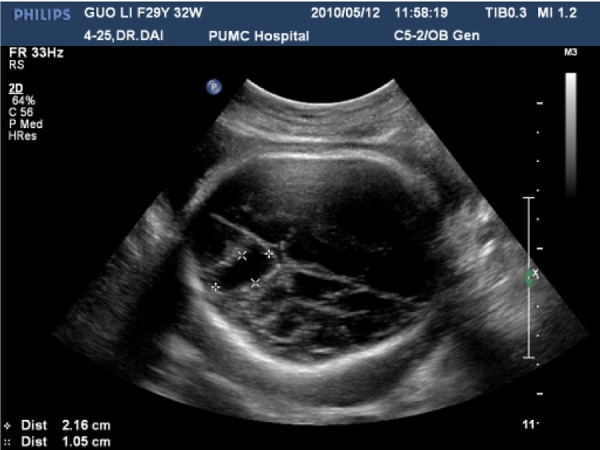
Ultrasound image (axial plane) of the fetal head at 32 weeks of gestation shows a cystic mass measuring 2.16×1.05cm at posterior fossa.
